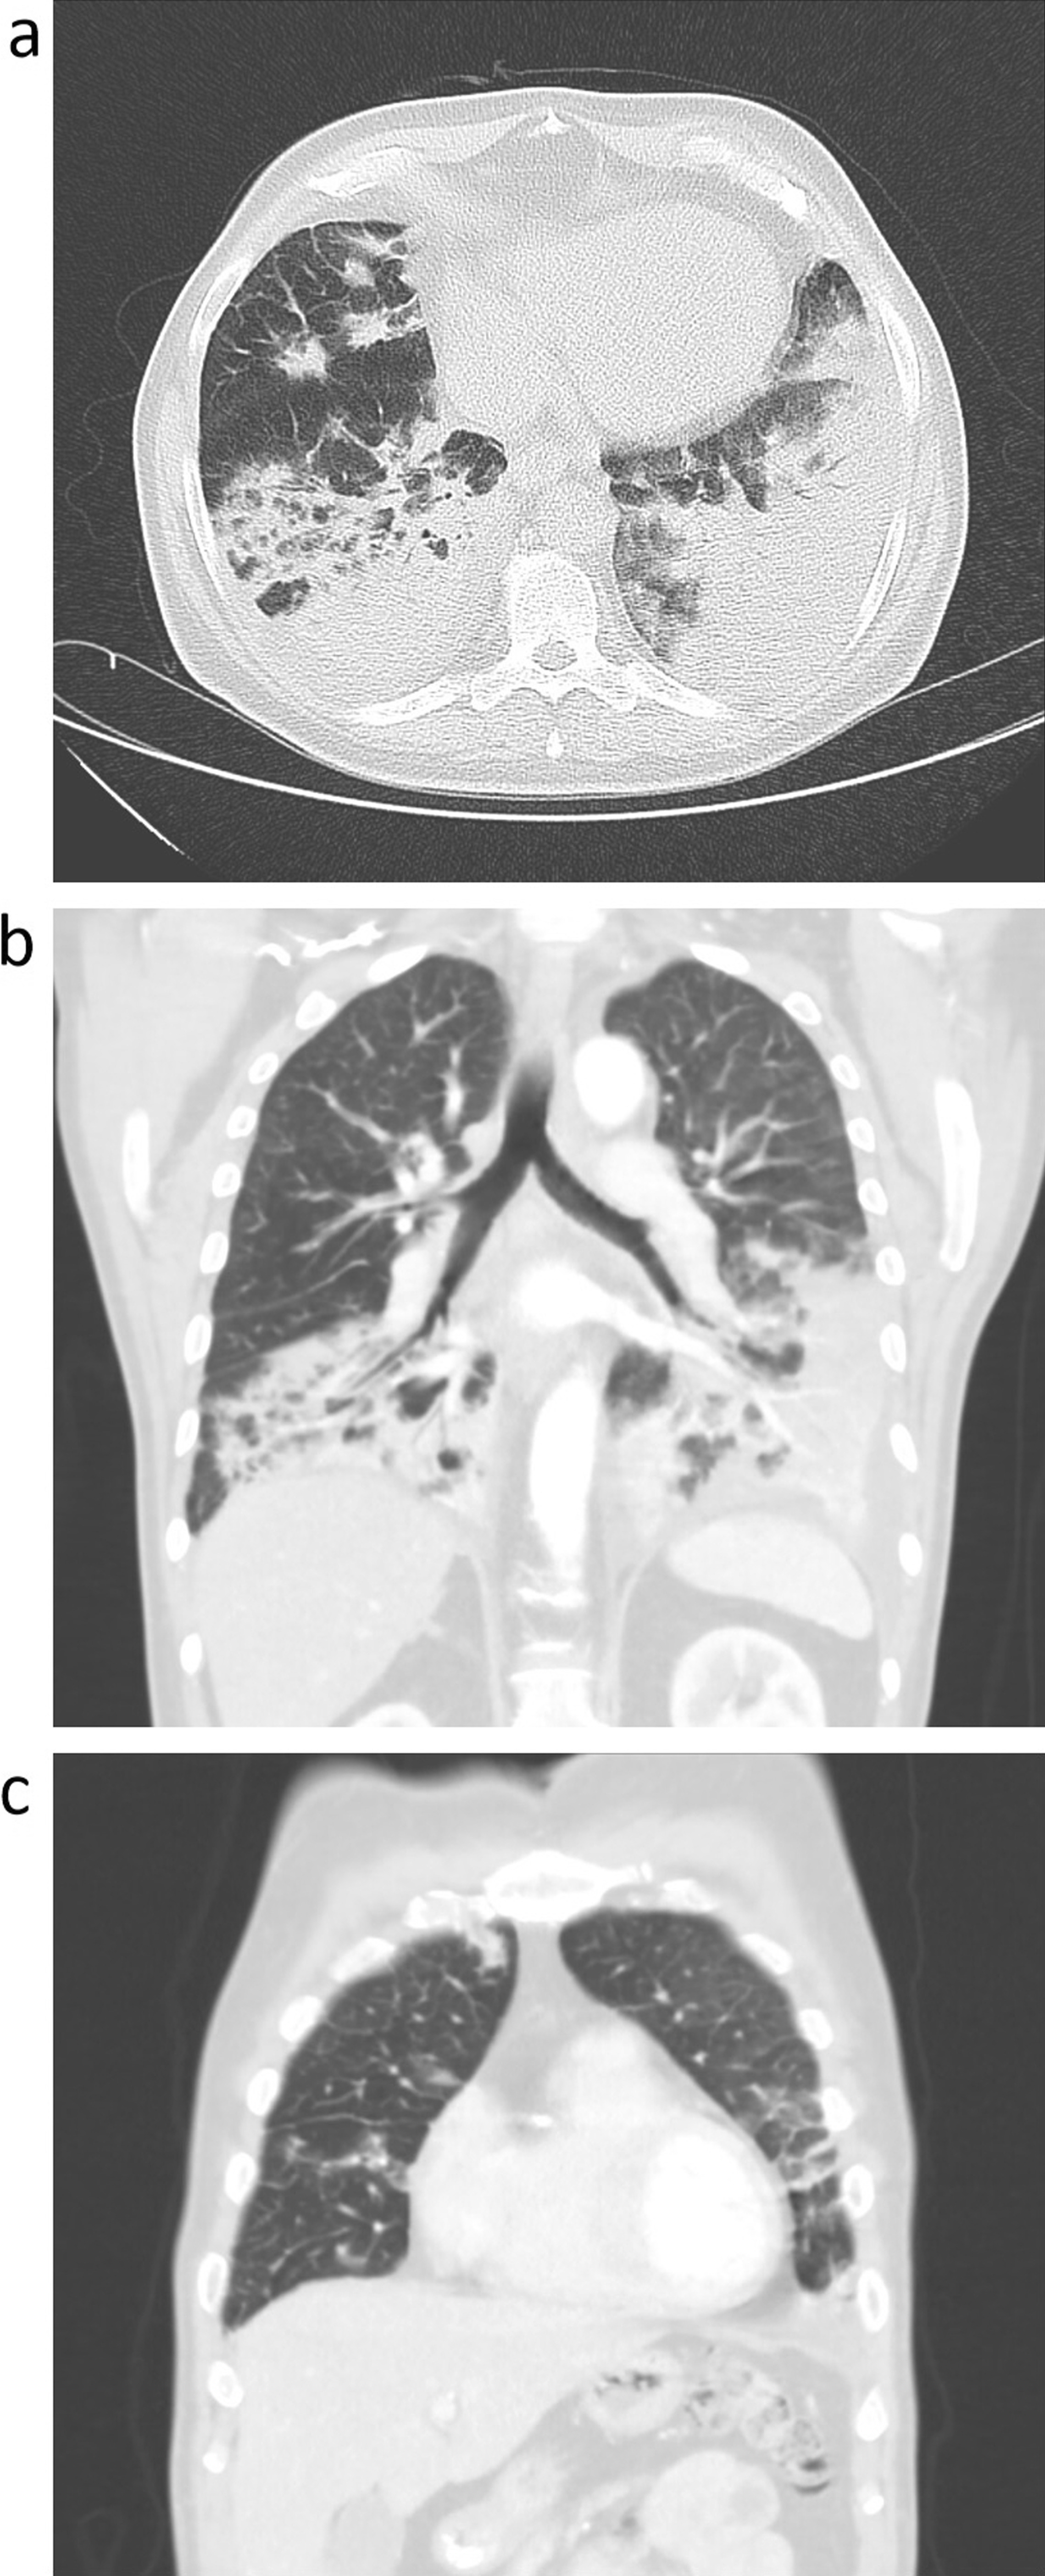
High-resolution computed tomography images: a computed tomography axial scan showing almost complete consolidation of both lower lobes; b computed tomography coronal scan showing almost complete consolidation of both lower lobes; c computed tomography coronal scan showing several smaller consolidations in the right upper and middle lobes and in the left upper lobe
